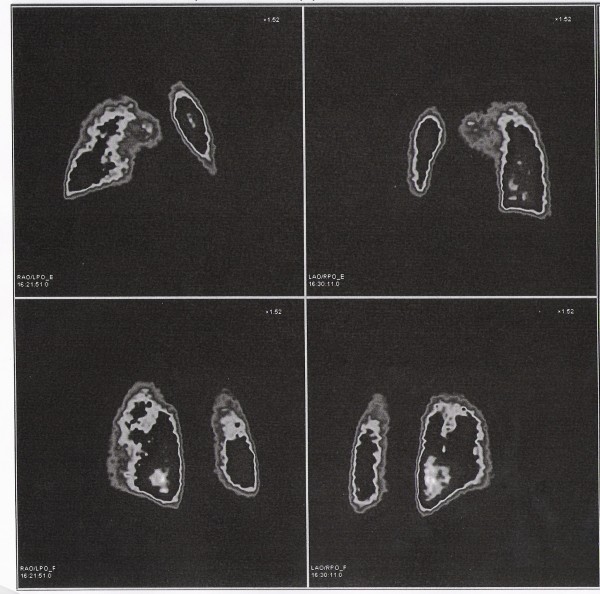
Lung perfusion scintigraphy shows the presence of multiple perfusion defects of a sub-segmental nature and bilateral distribution, that are particularly evident in the right superior lobe, suggesting an episode of bilateral pulmonary micropolyembolism.
